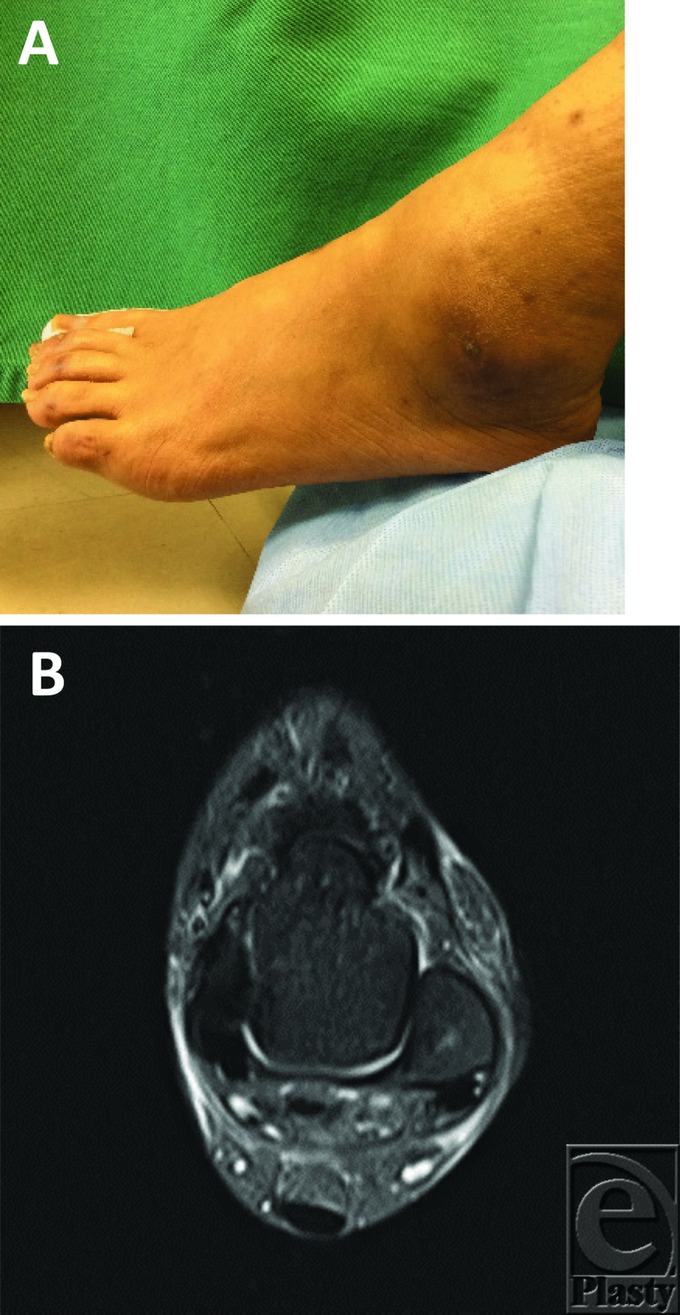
(a) Macroscopic finding showed that the orifice and the cavity were closed completely, and the skin color had improved 13 weeks after the first PRP therapy (3 weeks after the second PRP therapy). (b) Computed tomographic findings of the left lateral malleolus show that the cavity has disappeared.
